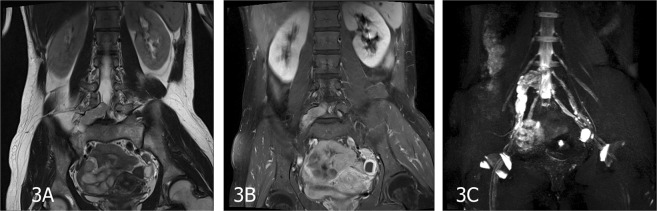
A 36-year-old female patient with a peripheral nerve sheath tumor in the right L5 spinal nerve. (A) On coronal T2-weighted images (T2WI), the mass shows short signal with ill-defined and irregular shape. (B) The tumor appears as a slightly hyperintense signal and was obviously enhanced on coronal enhanced T1-weighted fat-suppressed images. (C) Contrast-enhanced 3D-STIR SPACE with MPR image, the tumor was derived from the right L5 nerve, involving the lumbosacral trunk and from location along nerve distribution.
